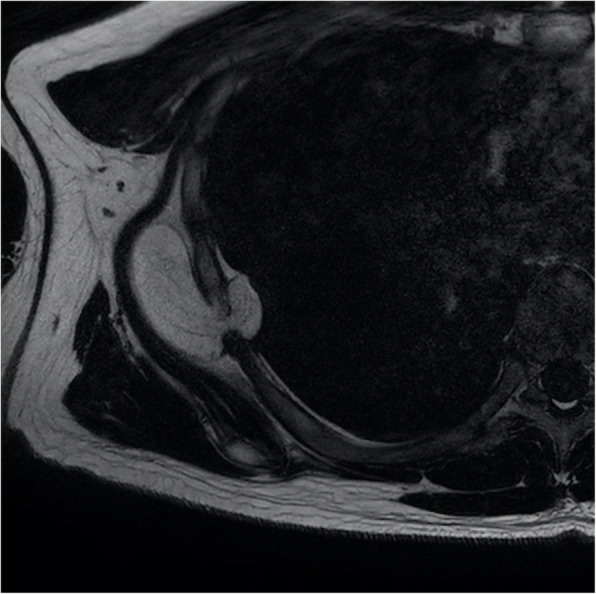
MR image revealing an encapsulated fatty mass. The mass was located between the right serratus anterior muscle and the right rib cage. The size of the mass was estimated to be 5.5 × 2.3 × 5.2 cm. The mass was insulated in the right 6th–7th intercostal space resulting in herniation into the pleural cavity
